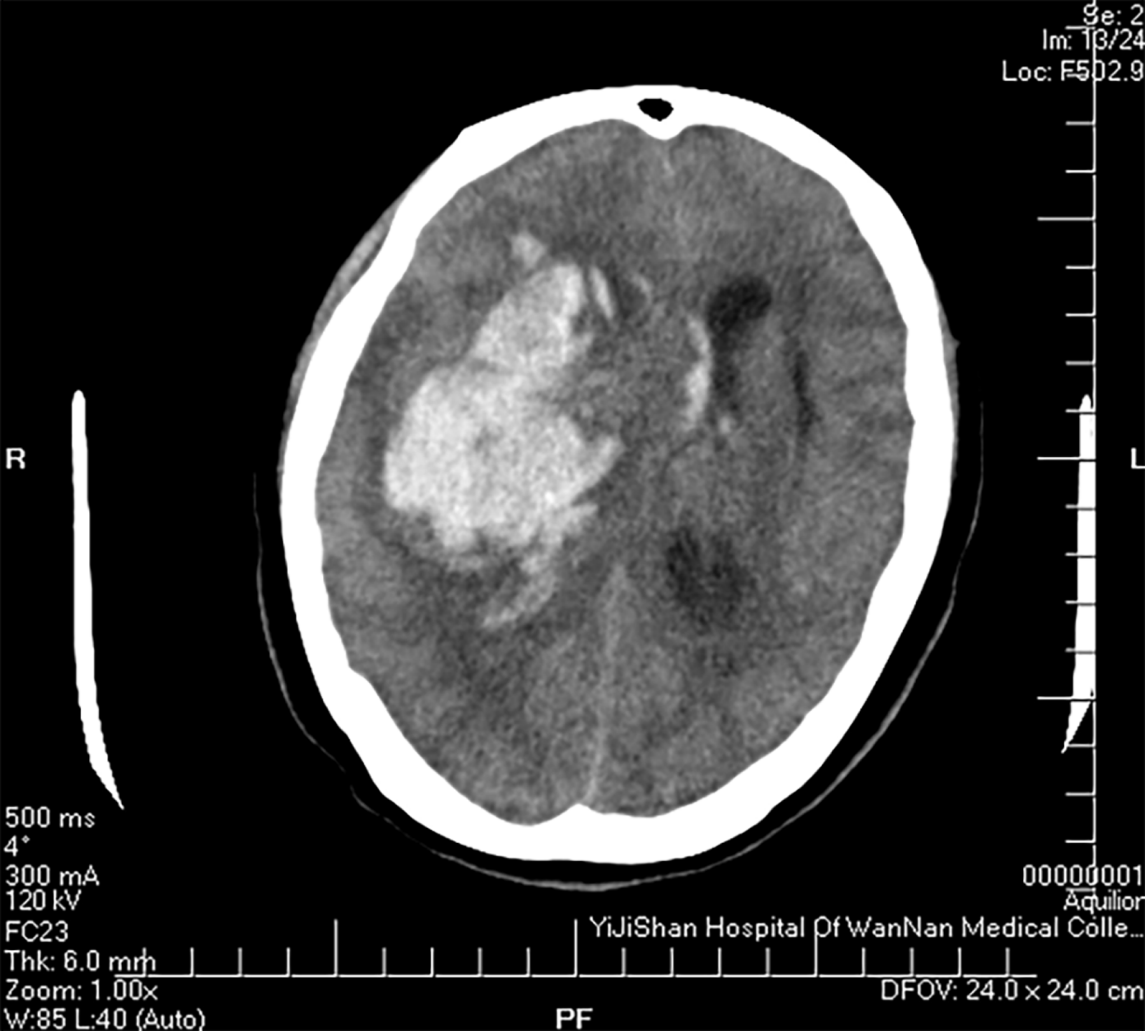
Cranial computed tomography scan showing right basal ganglia region cerebral hemorrhage
